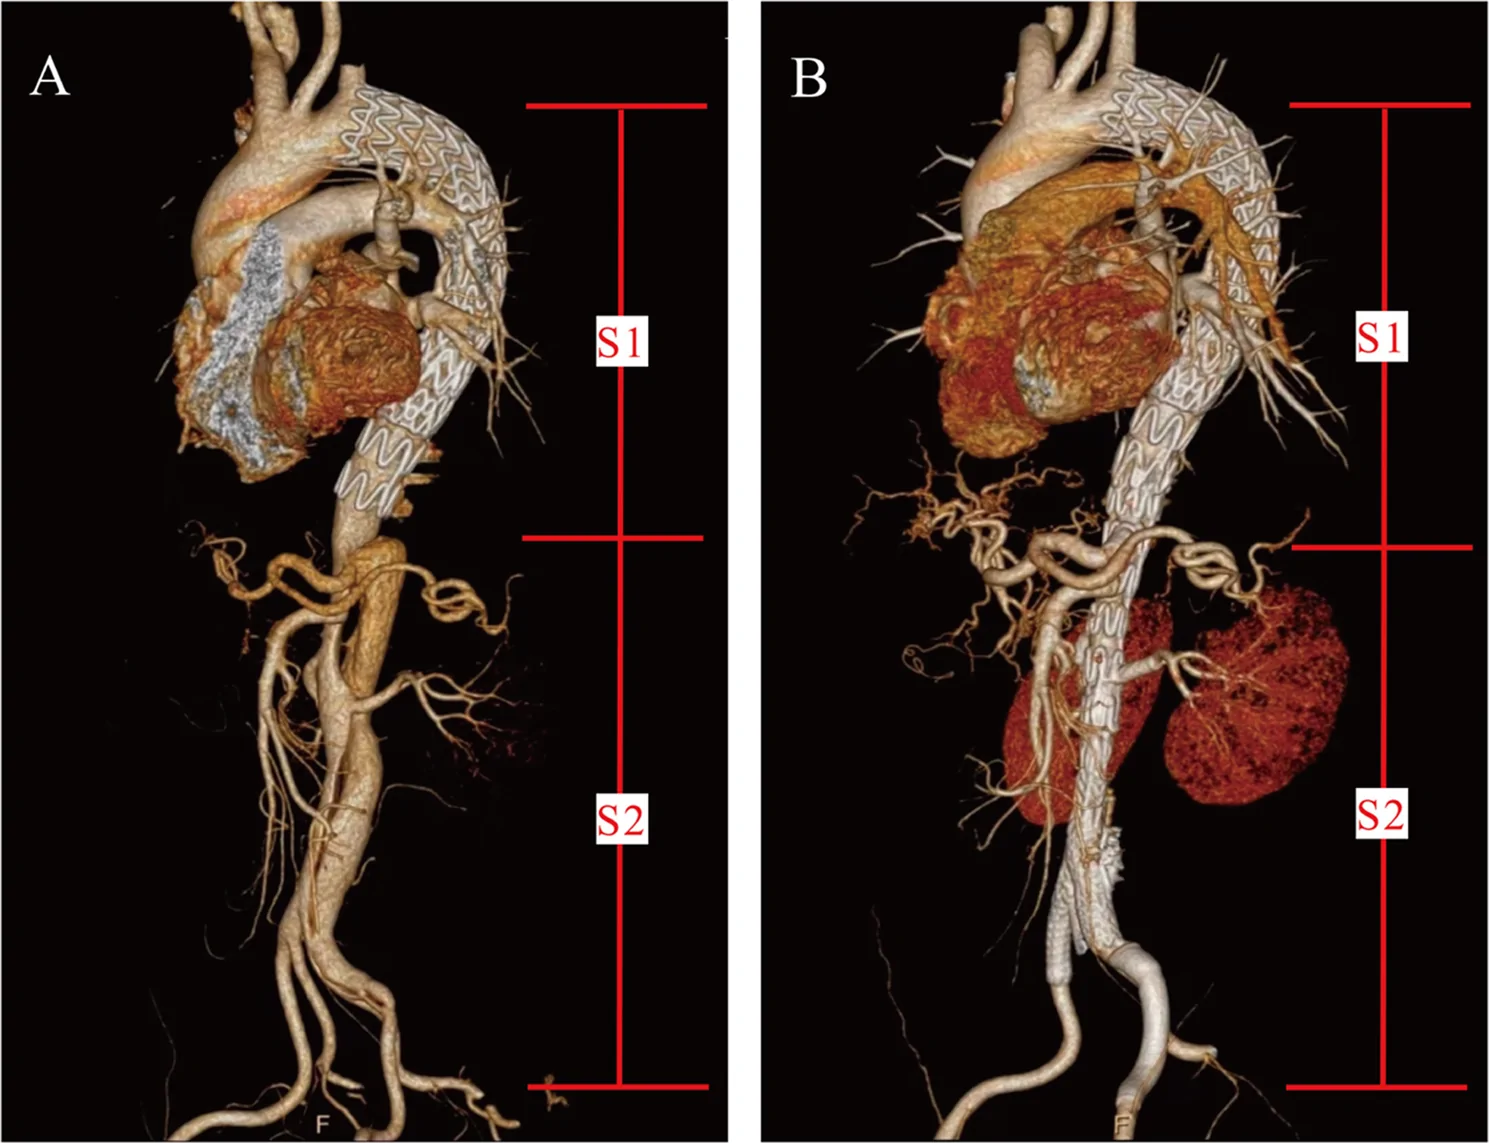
Computed tomography angiogram (CTA) of the patient before (A) and after (B) physician-modified stent grafts (PMEGs). S1, the stent-covered segment after thoracic endovascular aortic repair (TEVAR) surgery; S2, the distal residual dissection segment before the PMEGs procedure and the stent-covered segment after the PMEGs procedure
